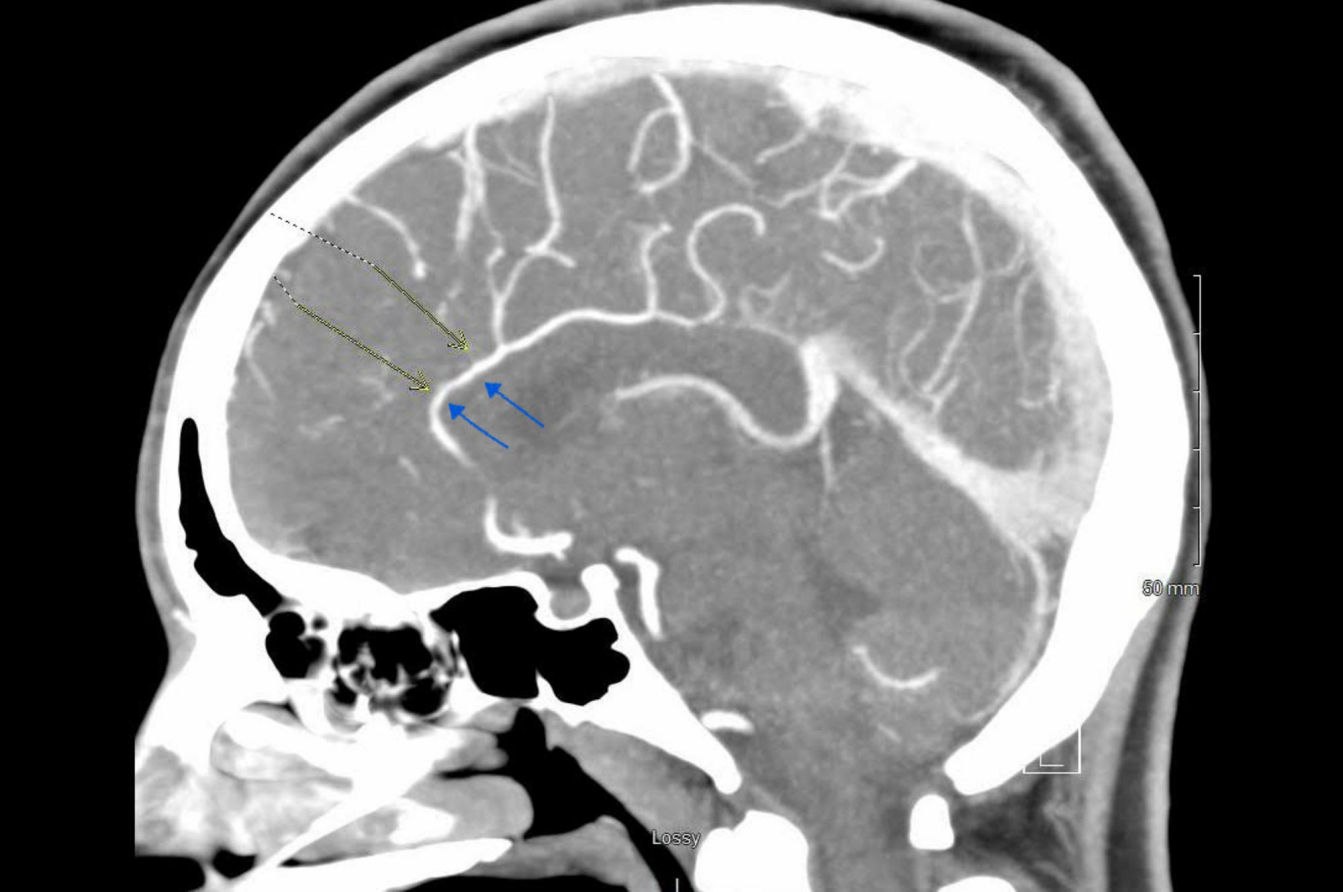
Sagittal section of CT angiogram brain showing the narrowing of the anterior cerebral artery CT: computed tomography
